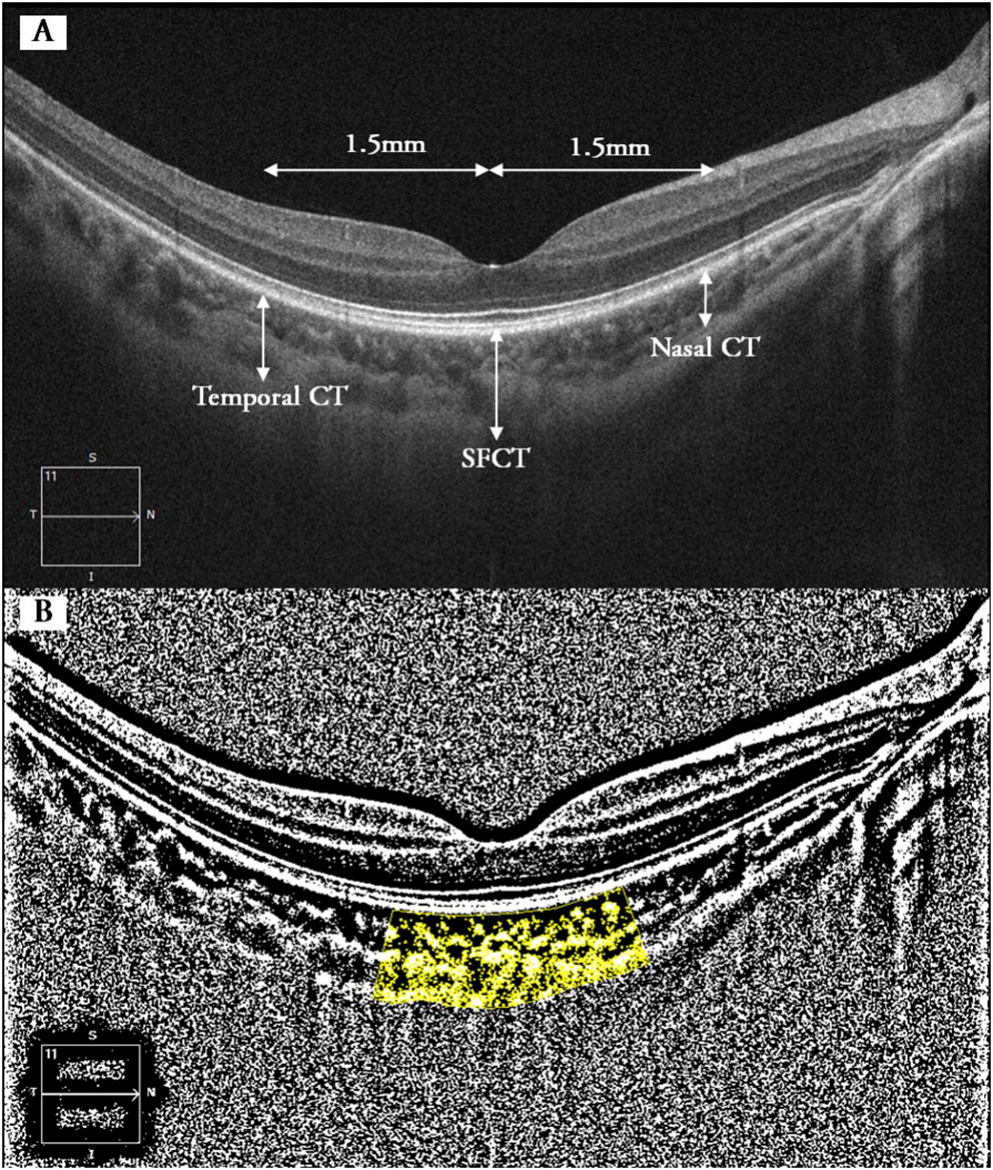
Binarization analysis of choroidal structure from OCT images. (A) Five locations where CT and CVI were measured (superior and inferior not shown). (B) Identification of the subfoveal choroidal segments using the binarization technique. Dark pixels refer to vascular components of choroid, whereas white pixels represent stromal components. CT, choroidal thickness; SFCT, subfoveal choroidal thickness; CVI, choroidal vascularity index.
